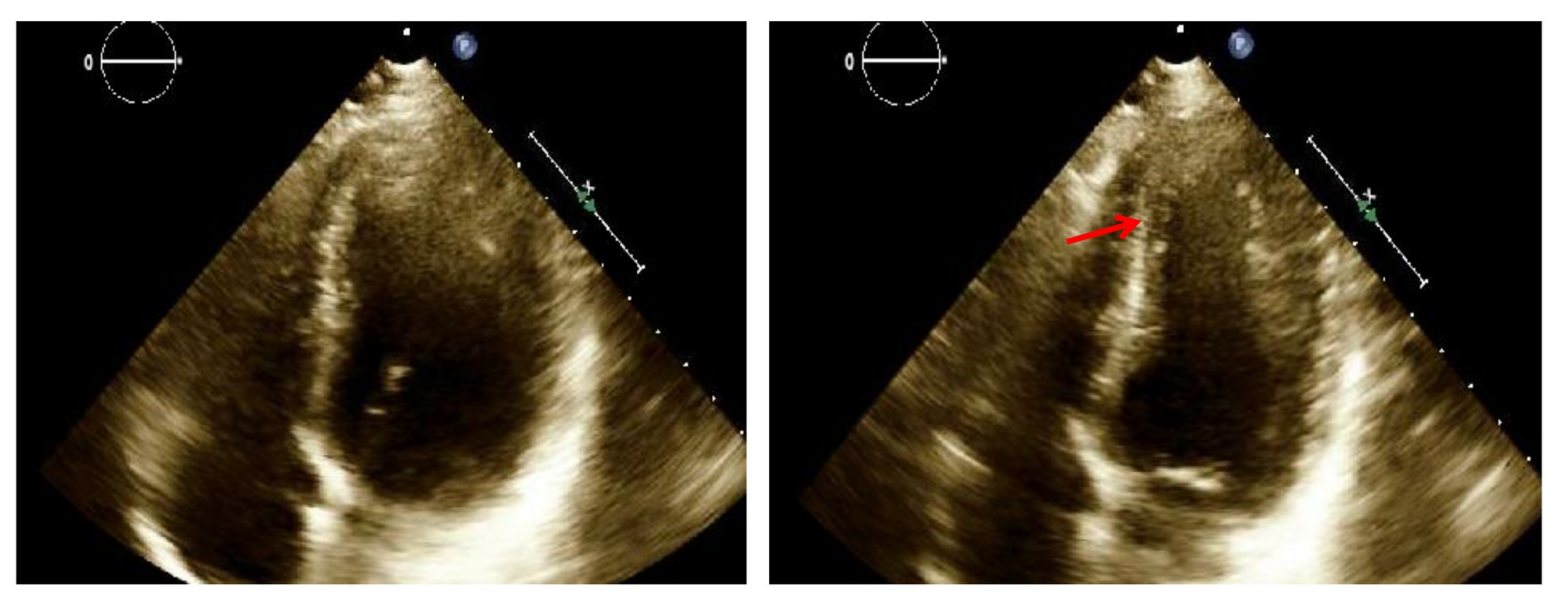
Transthoracic echocardiography on day 2 revealed regional wall motion abnormalities of the left ventricular apex with a low normal estimated left ventricular ejection fraction of 50%-55%. The right ventricle had normal systolic function and size. The left ventricle in four chamber view is shown in diastole (left) and systole (right) with an akinetic apex (arrow). No left ventricular thrombus was present.
